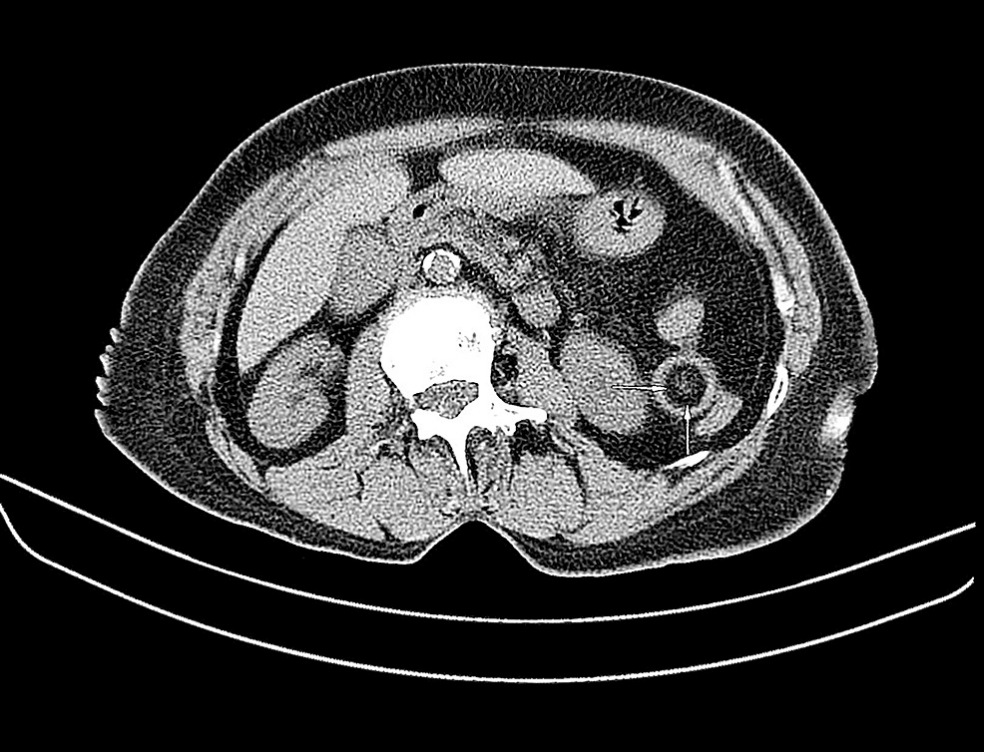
Axial non-contrast CT image through the upper abdomen demonstrating fat density (HU of - 85) within the lumen of the proximal jejunum (horizontal and vertical arrows), representing mesenteric fat
